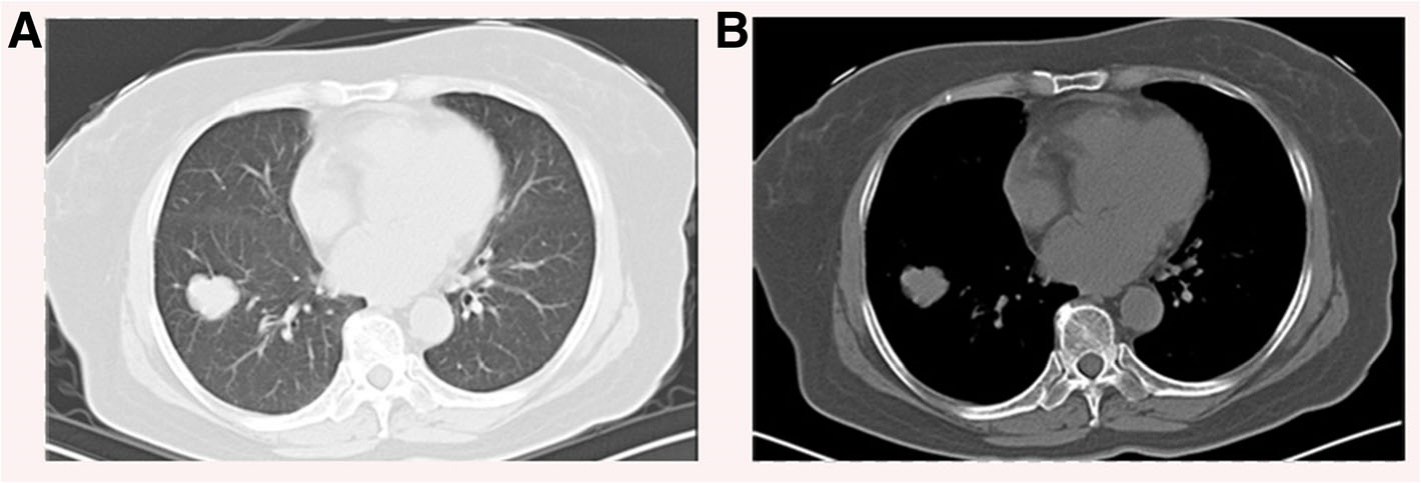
Chest CT scan showing a nodule in the right lower lung field. a Lung window. b Mediastinal window
